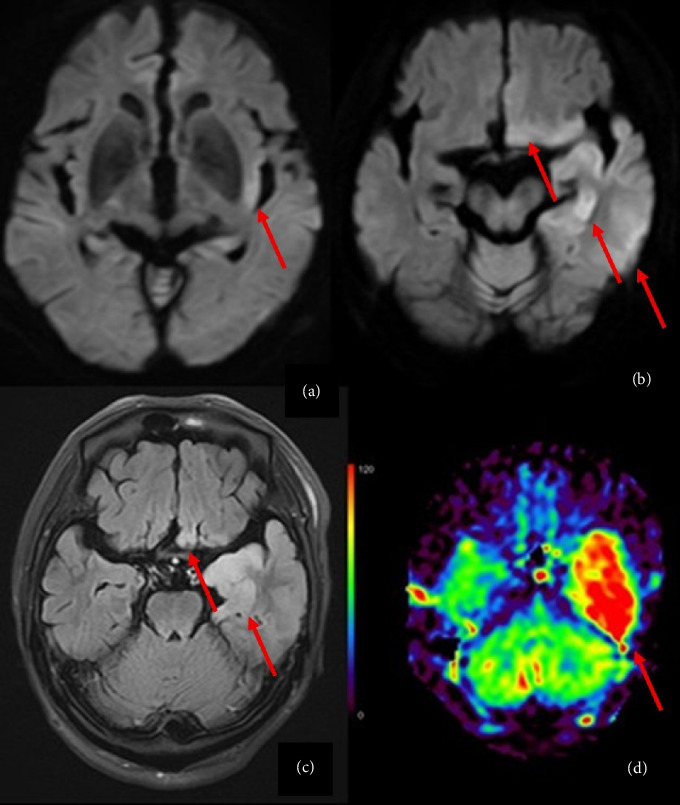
MRI on Day 1 and Day 4. (a) DWI on Day 1 showing a hyperintense region in the left temporal lobe. (b) DWI on Day 4 showing a high-signal region in the left temporal lobe, (c) FLAIR on Day 4 showing a high signal region in the left temporal lobe, and (d) ASL on Day 4 showing increased blood flow in the left temporal lobe. MRI: magnetic resonance imaging, DWI: diffusion-weighted imaging, FLAIR: fluid-attenuated inversion recovery, and ASL: arterial spin labeling.
